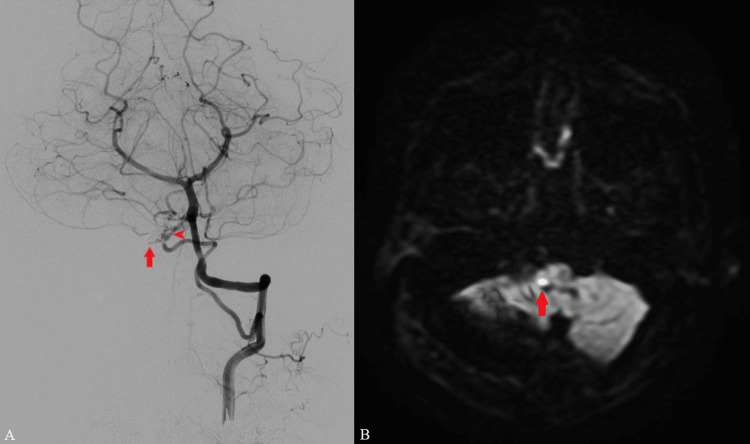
Postoperative left vertebral angiography and diffusion-weighted head magnetic resonance imaging. (A) Postoperative left vertebral angiography. Sacrifice of the vertebral artery-PICA aneurysm together with the right vertebral artery was performed (arrow). Right PICA was seen through the PICA-to-PICA anastomosis (arrowhead). (B) Diffusion-weighted head magnetic resonance imaging revealed a small infarction in the right medulla (arrow). PICA: posterior inferior cerebellar artery
